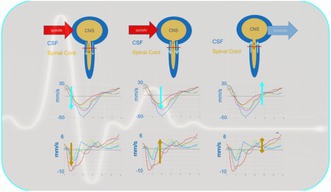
FIGURE 1 Example of cardiac dynamic phase‐contrast MRI measurements of CSF and spinal cord velocities.
